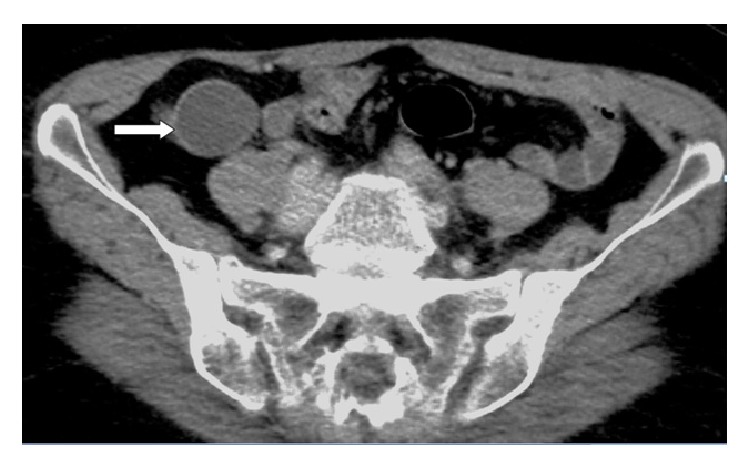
CT scan of the abdomen showing a mass in the right iliac fossa misdiagnosed as appendicular mass, white arrow (first case).
